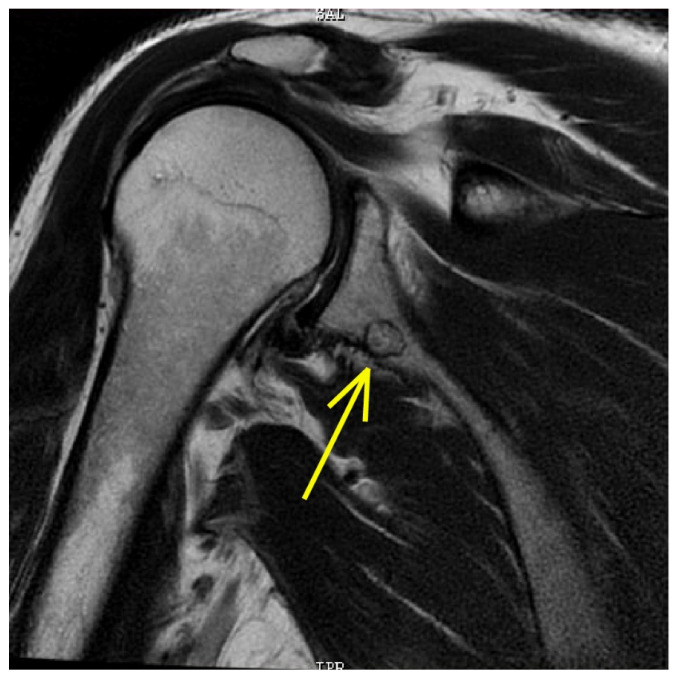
MRI scan. A roundish centimetric formation with a surrounding osteosclerotic border is visible at the lower portion of the scapular neck. This lesion has an uneven signal due to the presence of a central component with intermediate signal intensity (nidus) and is compatible in the first instance with osteoid osteoma with an atypical site (yellow arrow).
